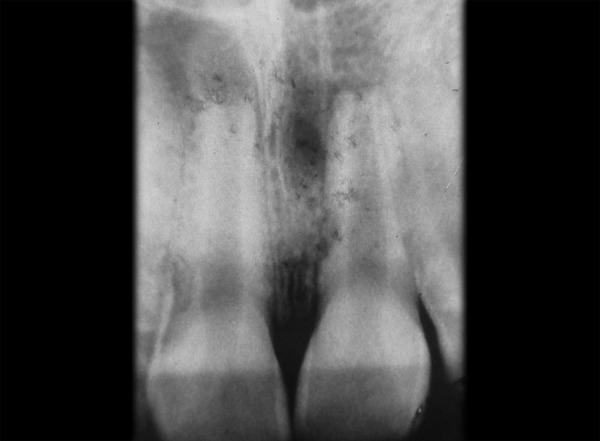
Maxillary central incisors of a 16-year-old female reveal signs of trauma induced external inflammatory root resorption in the right central incisor and apical resorption with periapical radiolucency with left central incisor
